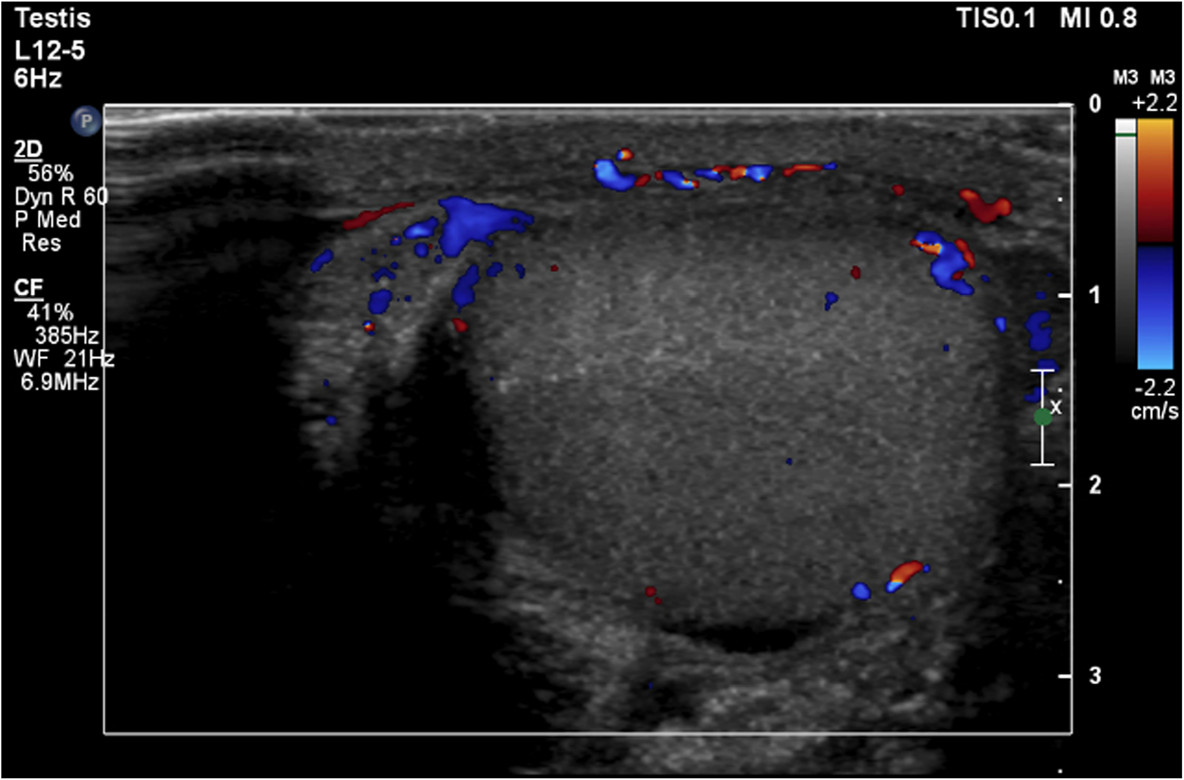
Color Doppler ultrasound of the left testicle demonstrating reduced vascularization of the left testicle
